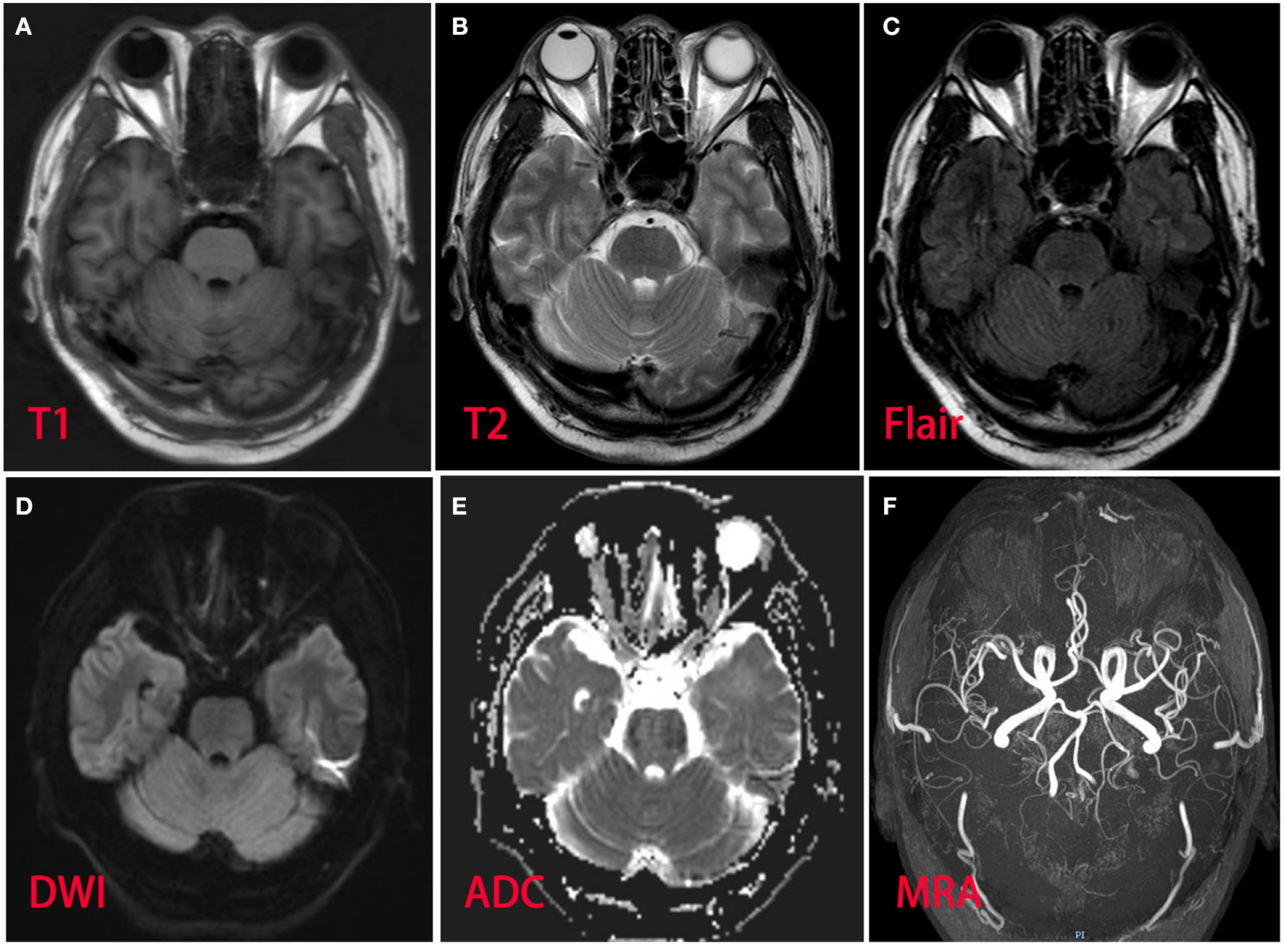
Magnetic resonance imaging and magnetic resonance angiography of the patient brain revealed normal. (A) T1 sequence; (B) T2 sequence; (C) fluid-attenuation inversion recovery (FLAIR) sequence; (D) diffusion-weighted (DWI) sequence; (E) apparent diffusion coefficient (ADC) sequence; (F) magnetic resonance angiography.
